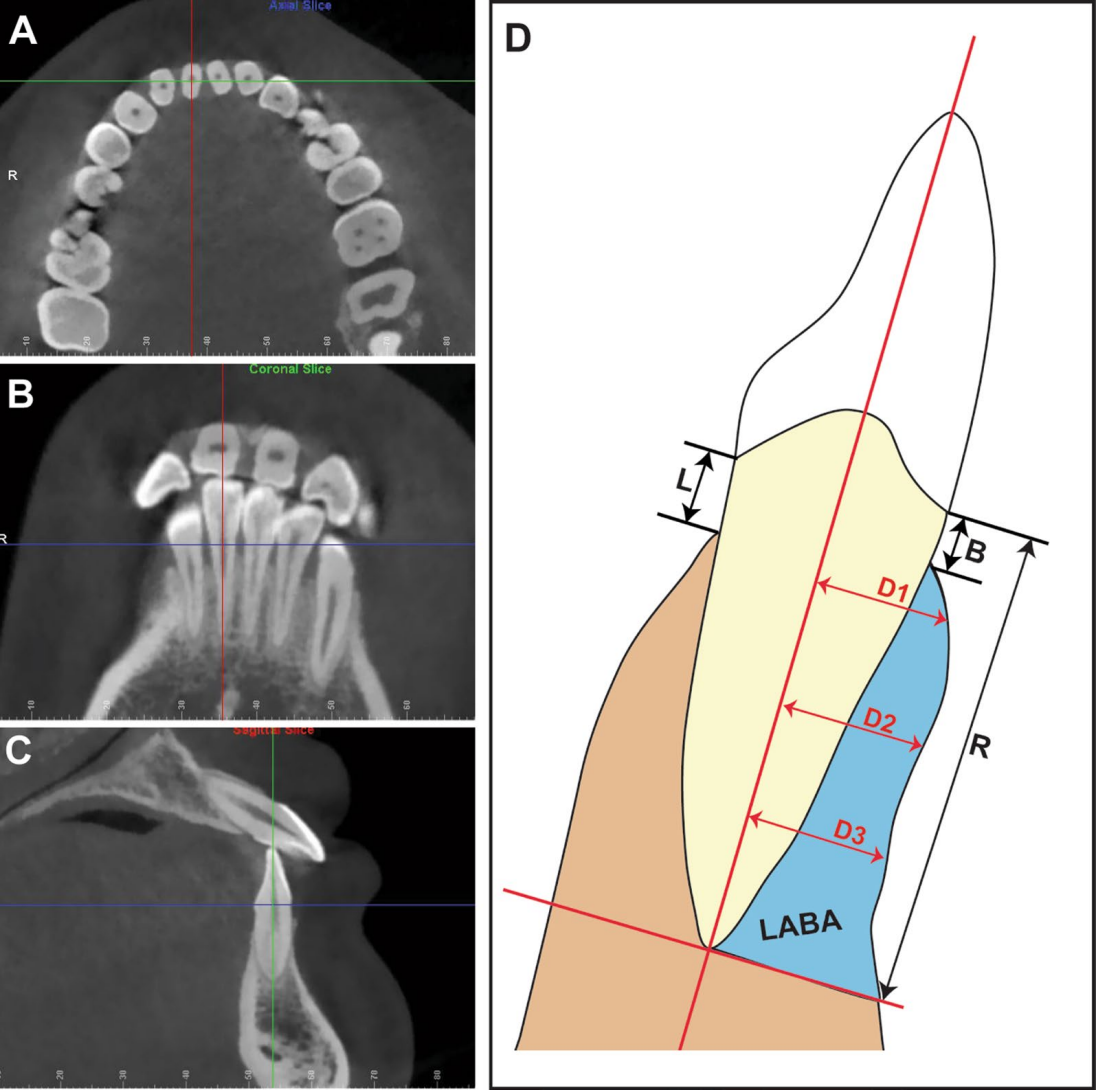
Representative images presenting the protocol of measurement. The correlated planes were determined by 3 intersected guidelines with different colors, which are blue for an axial plane, red for a sagittal plane, and green for a coronal plane. A Adjust the location of the axial plane by passing the blue guideline through the CEJ of the selected tooth in both the coronal and sagittal views, then rotate the green guideline until the intersecting line is the shortest. B Rotate the red guideline until it passes through the root apex and the midpoint of the incisal margin. C Rotate the green guideline until it passes through the root apex and the cusp. D Variables measured in CBCT in the sagittal plane. 1. LABA, the blue alveolar bone area of the labial side of the sagittal plane of the 6 mandibular anterior teeth. 2. vertical alveolar bone loss (CEJ-crest, the distance from the cementoenamel junction of the 6 mandibular anterior teeth to the alveolar bone crest measured on the sagittal plane); B, labial bone loss; L, lingual bone loss. 3. R, root length (distance from the cementoenamel junction of the 6 mandibular anterior teeth to the root apex, measured parallel to the long axis of the tooth on the sagittal plane); 4. upper (D1), middle (D2), and lower (D3) alveolar thicknesses (labial alveolar thickness on the sagittal plane as a distance from the root surface of the 6 mandibular anterior teeth to the labial surface of the alveolar bone, measured perpendicular to the long axis of the tooth at 3 mm, 6 mm, 9 mm below the CEJ)
